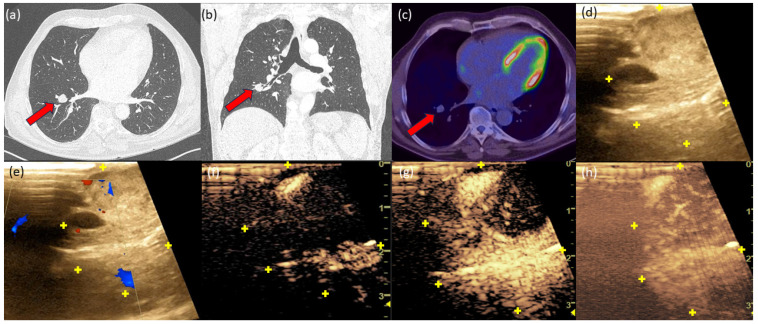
Chest CT-scan axial (a) and coronary (b) showed a clearly defined SPN with a diameter of 1.6 cm in the right lower lobe with an SUV of 13.7 in the FDG-PET/CT (c). The red arrow marks the tumor. In B-mode (d), we see a partially sharply demarcated, echo-heterogeneous tumor. The yellow “+” marks the tumor margin. In CCDS (e), a peripheral vessel section is distinguishable apically at the tumor margin and centrally. In the early contrast agent phase (f) at t = 12 s, a clear central and peripheral contrast can be seen, which rapidly spreads over the tumor (g) at t = 18 s. (h) The 3D reconstruction of the tumor.
